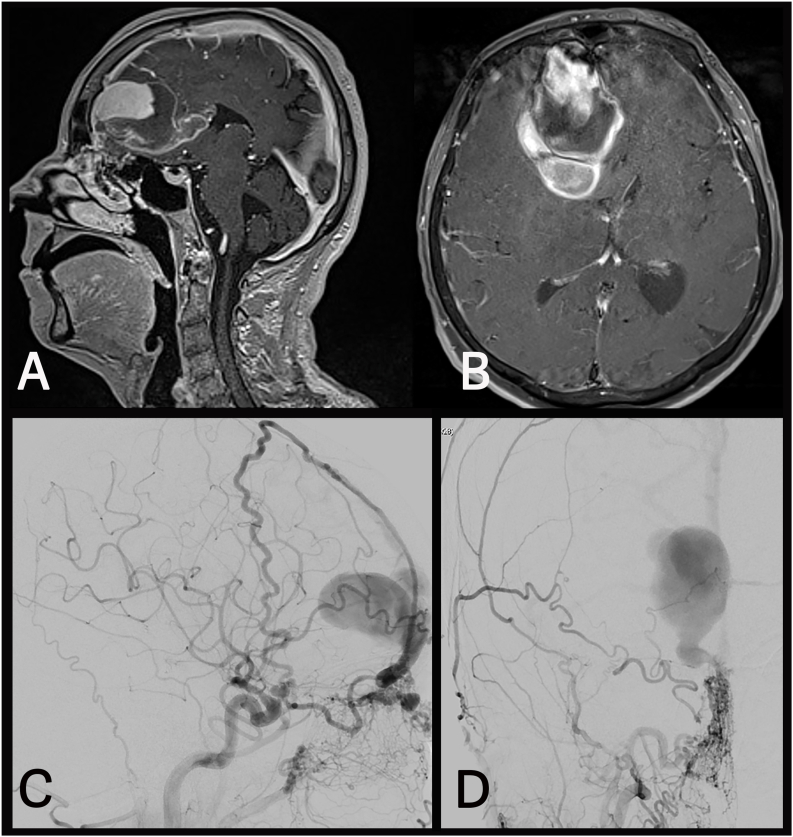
(A) and (B) show preoperative MR images of the ethmoidal fistula with a large venous aneurysm. The initially suspected intracerebral hemorrhage (ICH) posteriorly to the aneurysm, was intraoperatively identified as a thrombosed component of the venous outpouching. (C) and (D) show preoperative lateral and an anteroposterior (A.P.) projections of the fistula with feeders originating from the ethmoidal arteries.
